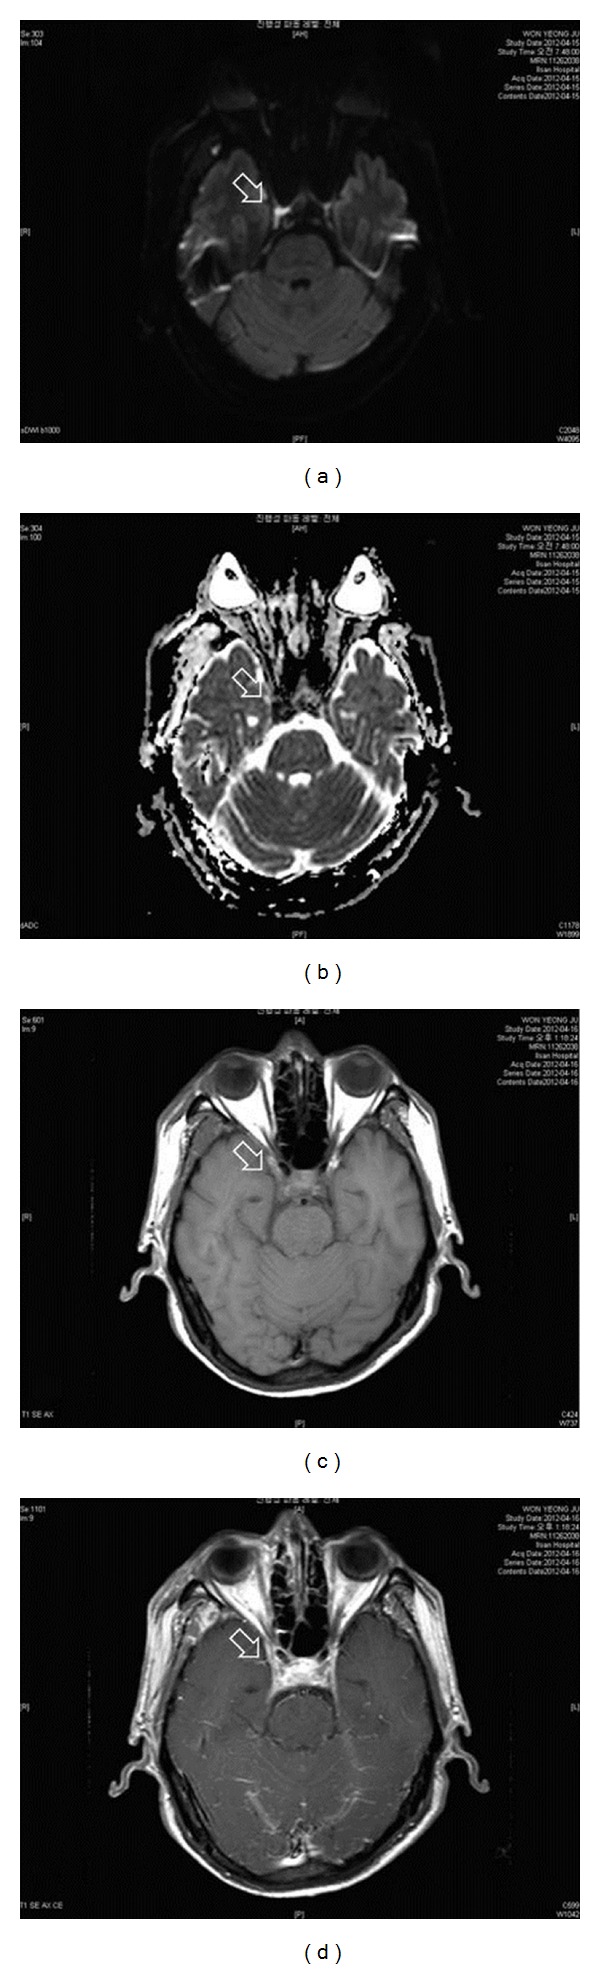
MR images reveal the presence of an abscess in the cavernous sinus area (white arrow, (a)–(d)). Diffusion restriction around the right cavernous sinus indicates an abscess in the diffusion-weighted MR image (a). There is no high signal around the right cavernous sinus in the apparent diffusion coefficient map (b). Precontrast T1-weighted MR image (c). More enhancement around the right cavernous sinus area was observed in the postcontrast T1-weighted MR image (d).
